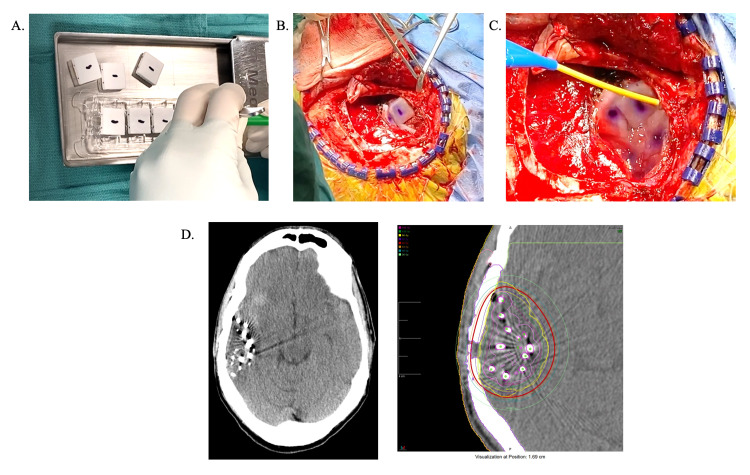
GammaTile Implantation A. GammaTiles pictured here with Cs tiles embedded in the collagen matrix. When implanted, they are inserted with the rough side down. B. After hemostasis is achieved and the resection cavity is irrigated, GammaTiles line the cavity without stacking. C. Fibrin glue can be used to secure the tiles, particularly in larger resection cavities or if there is concern about the mobility of the tiles. D. GammaTiles can be visualized on postoperative CT scan to ensure the cavity is lined and for dosimetry calculations.
